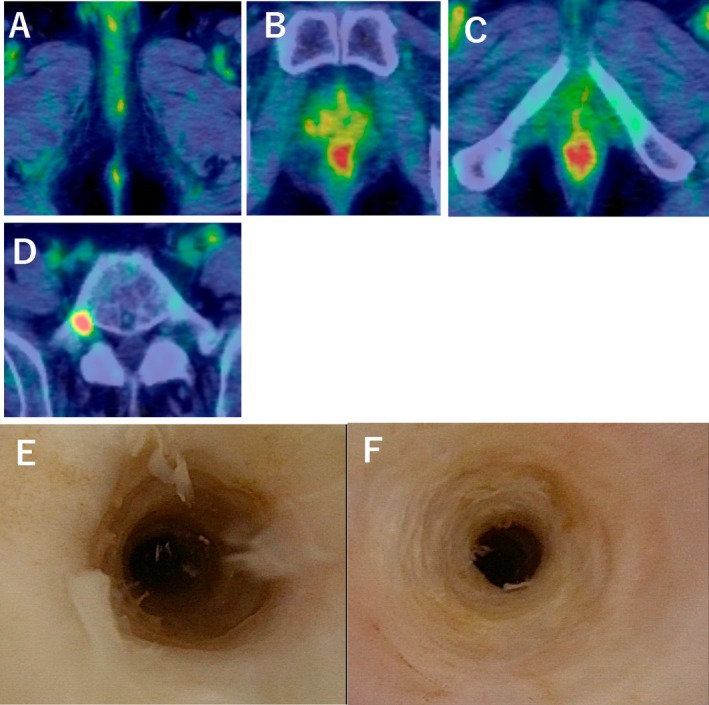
Clinicopathological findings after chemoradiotherapy. (A–C) FDG‐PET‐CT showing reduced FDG uptake in the prostate (A), urethra (B), and ischium (C). (D) New FDG uptake in the fifth lumbar (L5) vertebra. (E, F) Follow‐up urethroscopy showing no residual tumor in the pendulous urethra. FDG‐PET‐CT, 18F‐fluorodeoxyglucose positron emission tomography–computed tomography.
